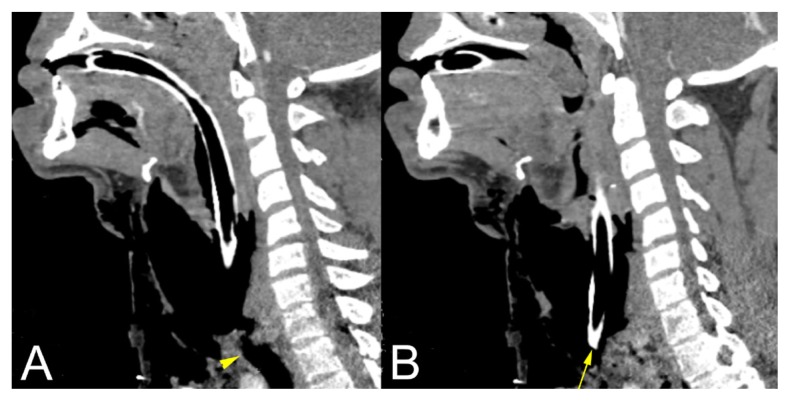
Sagittal images of a CT of the neck show the ET tube location relative to trachea: A) note the location of the trachea and trajectory of the ET tube, B) the ET tube tip lies anterior to the trachea.
